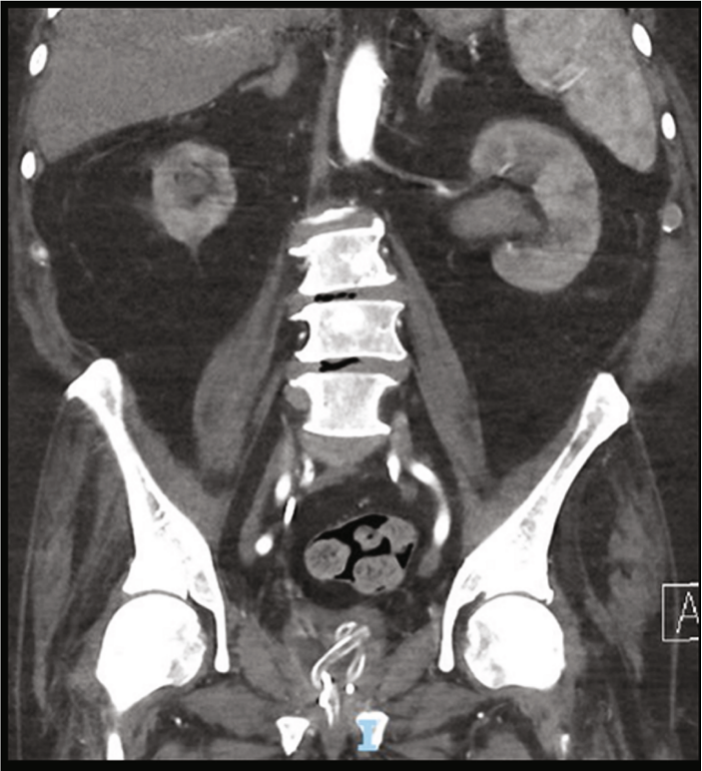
Coronal CT demonstrating knotted catheter and right ureteric stent.
